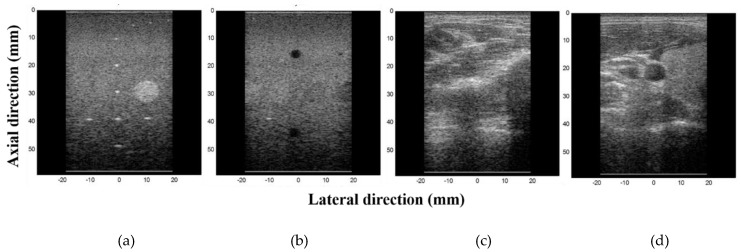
Ultrasound images showing (a) point targets, (b) cysts, (c) nerve and (d), which were constructed by utilizing the proposed BL code.
